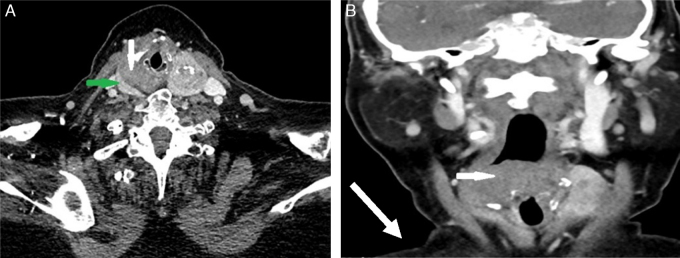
CT scan, with contrast, of the neck. (A) Axial view at C5/6 showing the enhancing right thyroid lobe (green arrow) and the non-enhancing mass arising from the hypopharynx (white arrow). A plane of separation is clearly visible in between. (B) Coronal view of the hypopharyngeal mass (white arrow).
